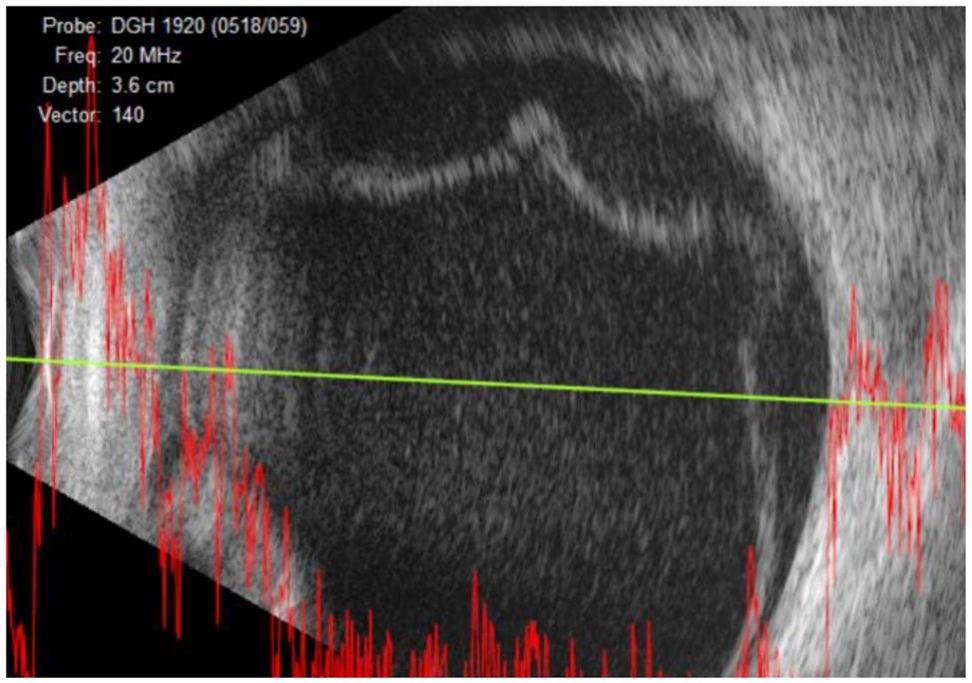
B-scan Ultrasound of the left eye six days after consultation at our hospital
